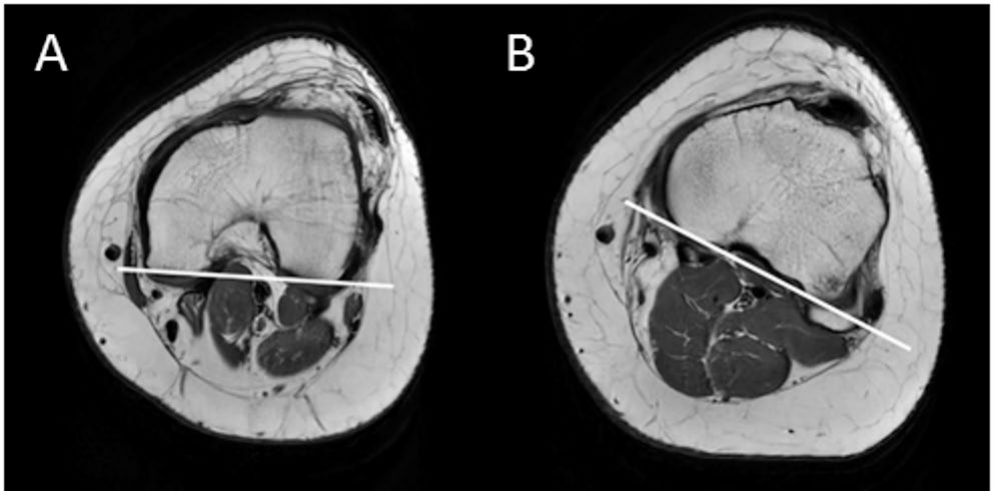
Torsional MRI of a left knee. Drawn lines showing the dorsal femoral condyle tangent line (A) and the dorsal tibial head tangent line (B). The angle between the two tangents is the TF rotation
